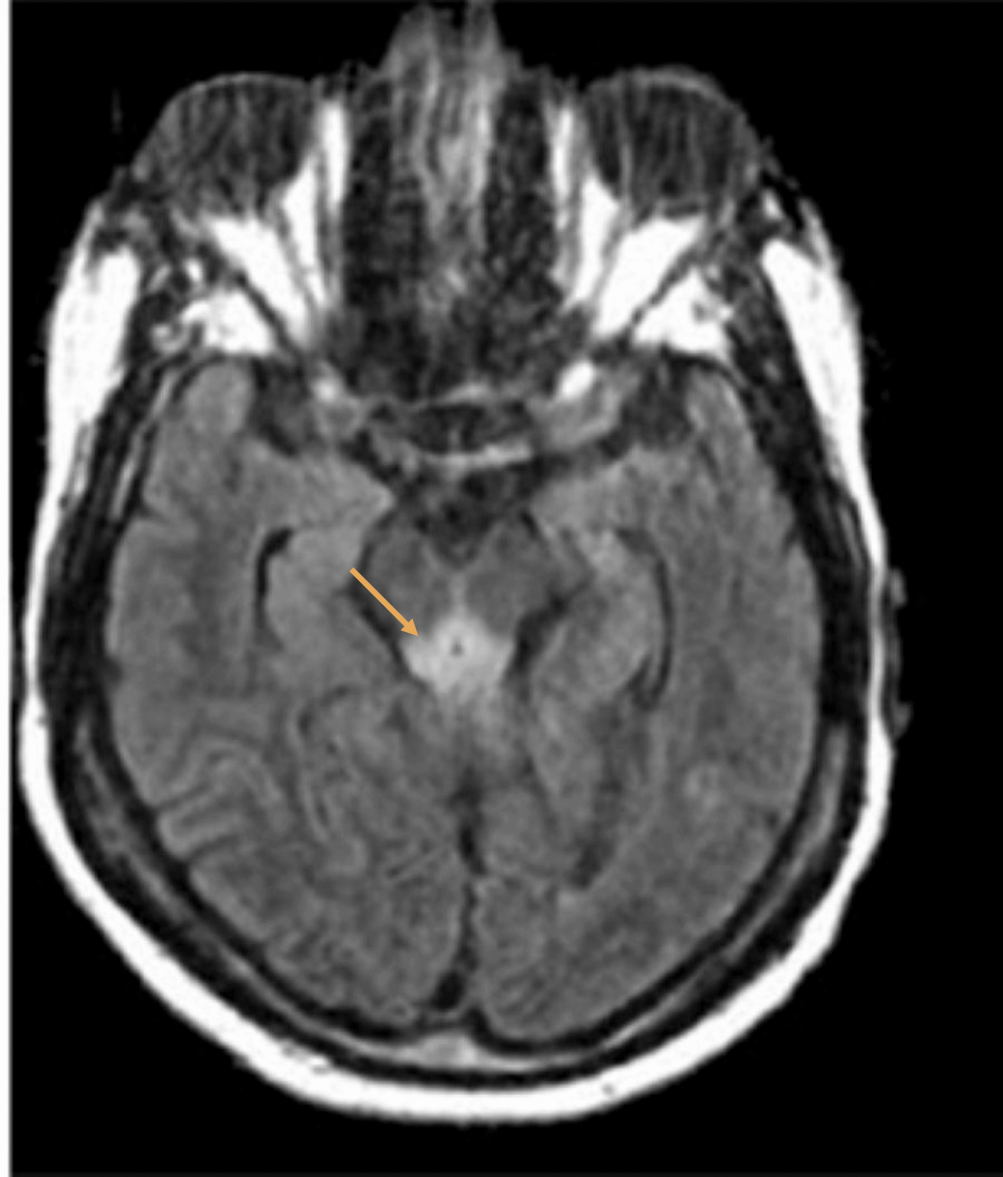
Magnetic resonance imaging of the brain revealing symmetrical abnormal fluid-attenuated inversion recovery hyperintensity within the periaqueductal gray matter.
